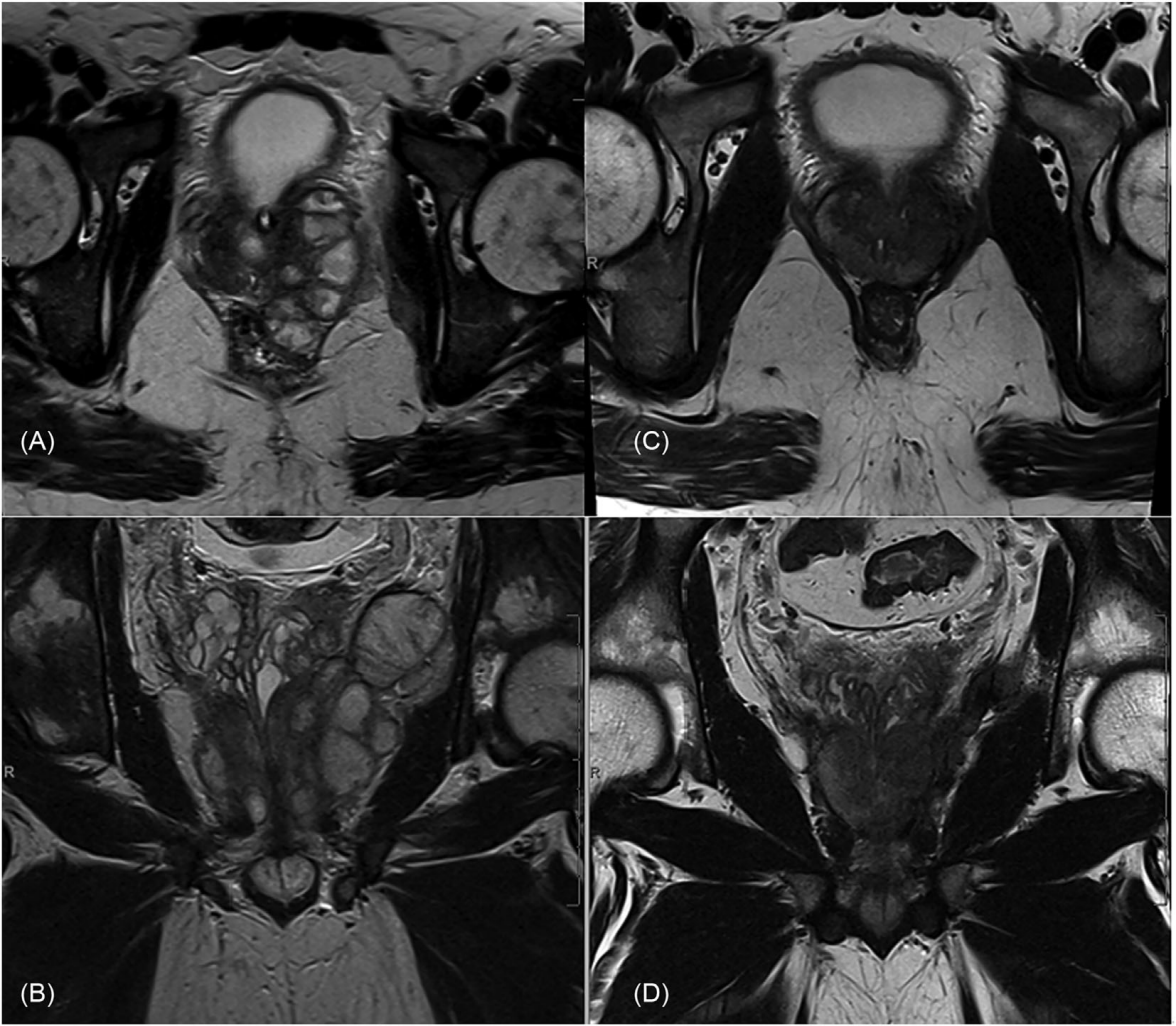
MRI images showing the prostate abscess for Case 1, a 33‐year‐old male. (A) Axial view and (B) coronal view are images obtained at diagnosis. Note how the collection extends into the left pelvic wall. (C) Axial view and (D) coronal view were obtained at 7 weeks post‐drainage and show near complete resolution of the abscess.
